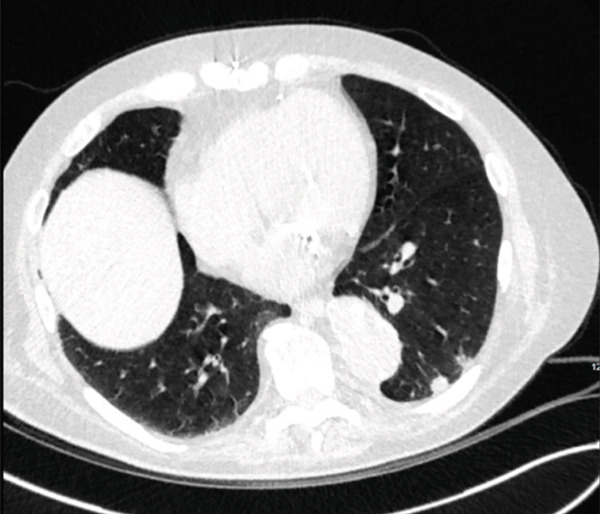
Noncontrast chest CT on April 11, 2023 showing an irregular nodule in the right lung apex measured 13 × 10 mm.
